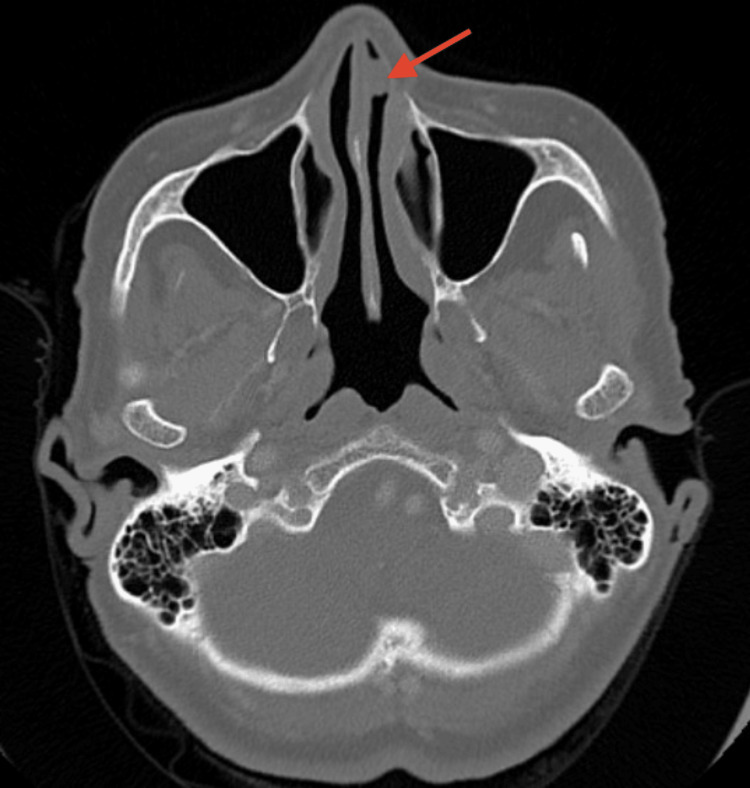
Axial CT scan revealing the presence of an 8x8 mm mass in the left nasal cavity CT, computed tomography
